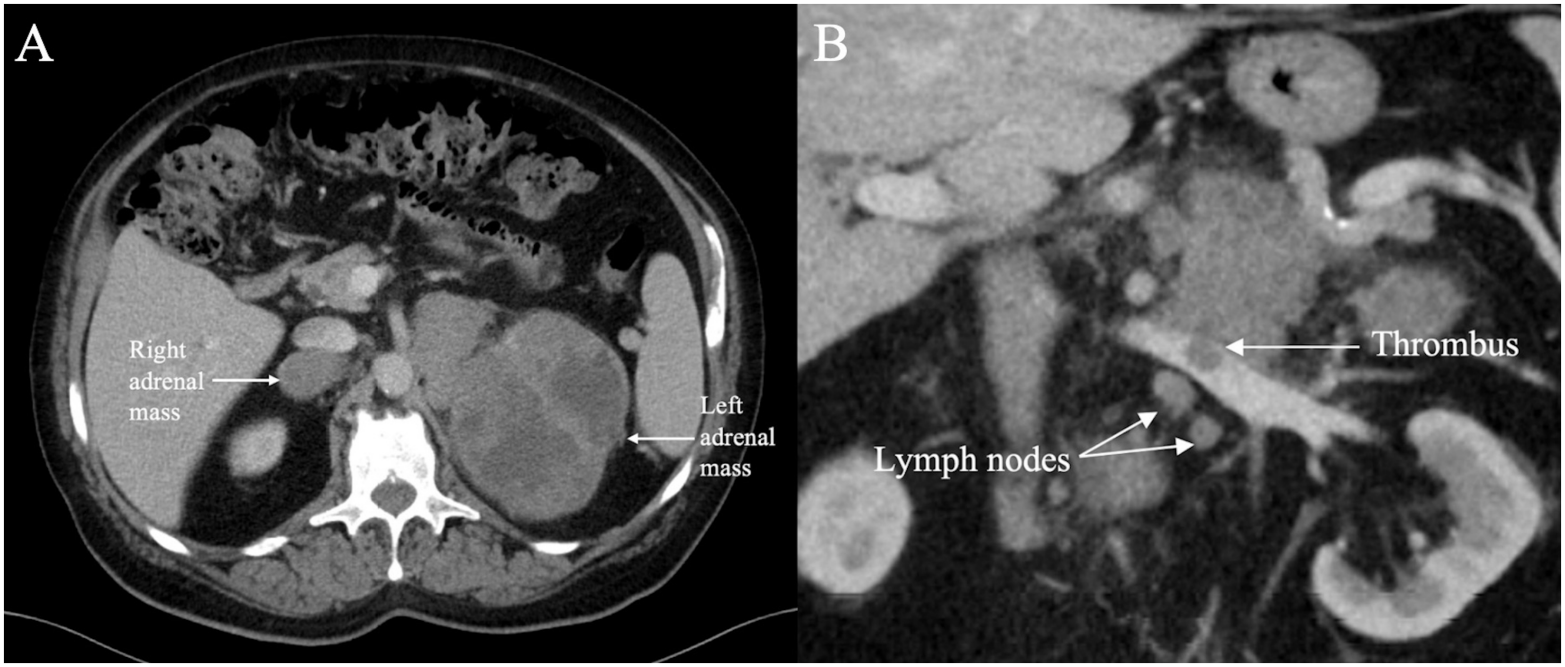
Axial contrast enhanced CT (A) through the upper abdomen in November 2020 shows a 11 x 9.9 cm lobulated heterogeneously enhancing solid cystic mass in the left adrenal gland and a 4.6 x 3.4 cm similar appearing mass in the right adrenal gland (indicated by arrows). Coronal contrast enhanced CT (B) through the upper abdomen in November 2020 shows an intraluminal filling defect in the left renal vein consistent with thrombus associated with the left adrenal mass. A few suspicious lymph nodes are present in the vicinity.
